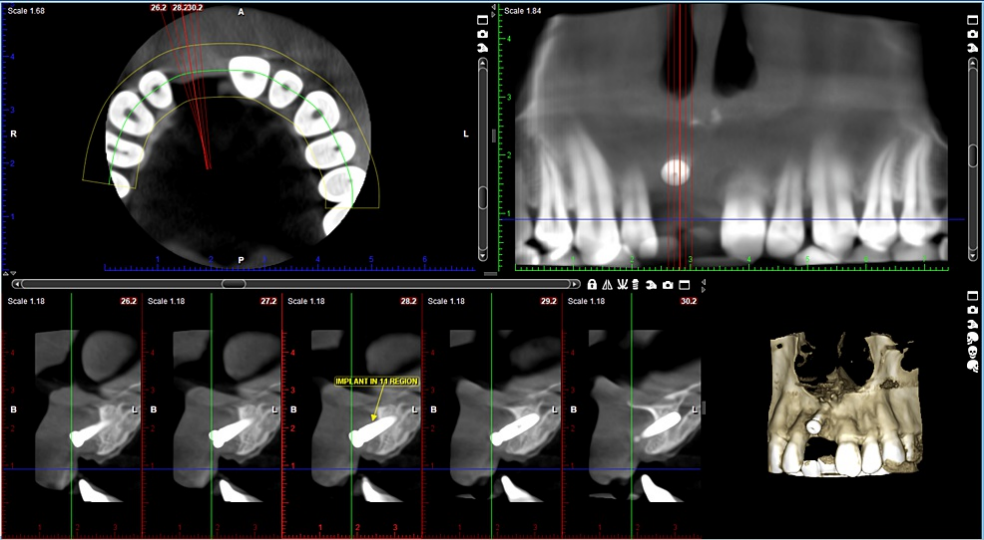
CBCT showing malpositioned implant in 11 region CBCT: cone beam computed tomography Image Credit: Ankita Pathak
